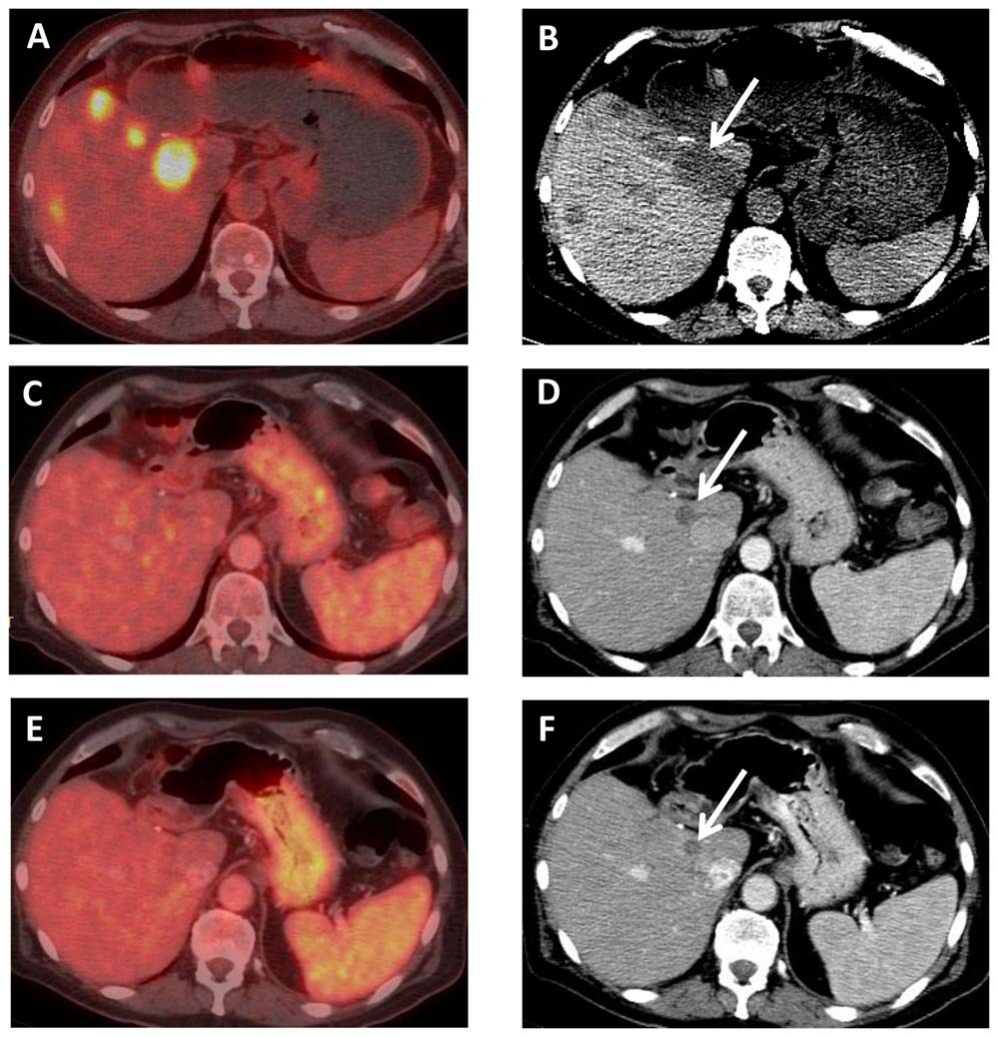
A 67 year old male with *BRAF* mutated intrahepatic CCA, who had progressed on conventional chemotherapy. Axial (A) fused PET-CT and (B) unenhanced CT images from a PET scan demonstrate FDG avidity of multiple liver metastases. After 8 weeks of BRAF inhibitor therapy, axial (C) fused PET-CT and (D) contrast-enhanced CT images demonstrate lack of FDG avidity and decreased size of liver metastases, e.g., the dominant lesion adjacent to the IVC (arrow) decreased from 3.7 cm to 1.6 cm. After 16 weeks of therapy, axial (E) fused PET-CT and (F) contrast-enhanced CT images demonstrate continued lack of FDG avidity and further decreased size of liver metastases, e.g., the dominant lesion (arrow) now measures 1.3 cm.
